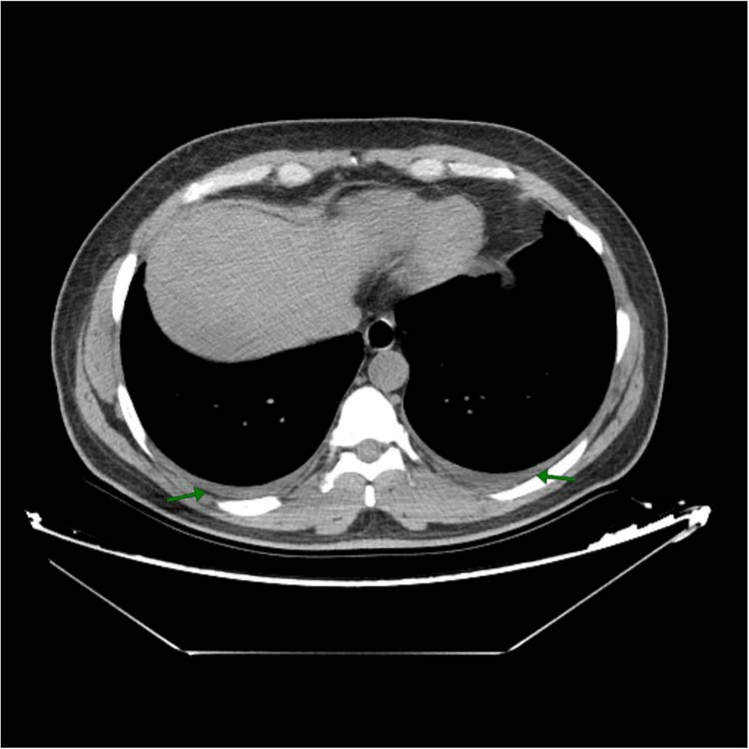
Axial section of native chest CT scan shows bilateral mild pleural effusion with arrows indicating the affected areas.
